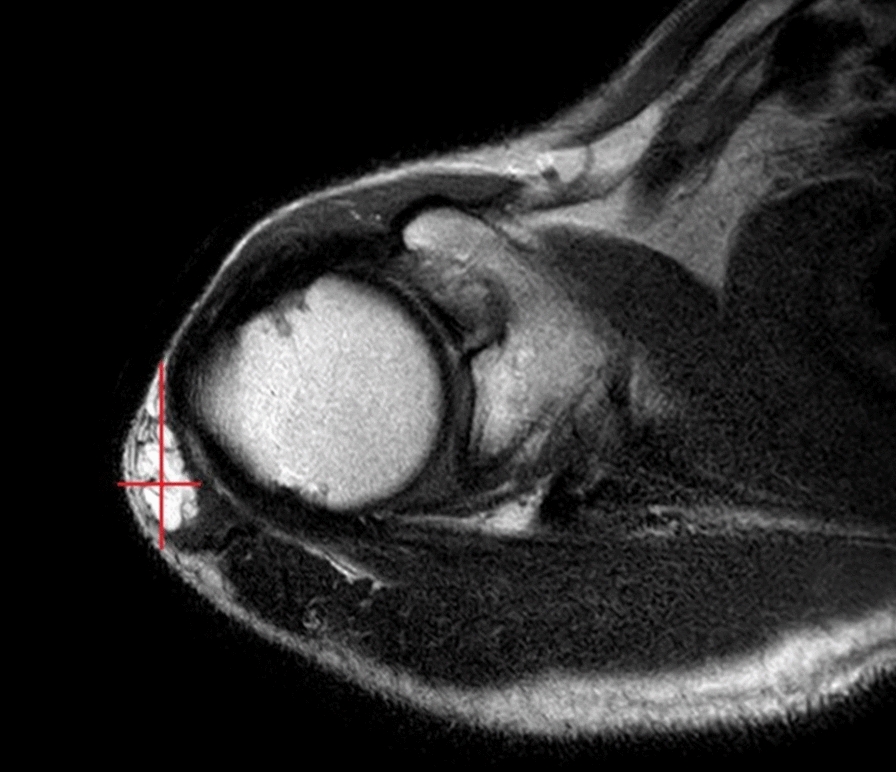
MRI at recurrence presentation, showing a 35 × 15 mm mass within the right deltoid muscle
